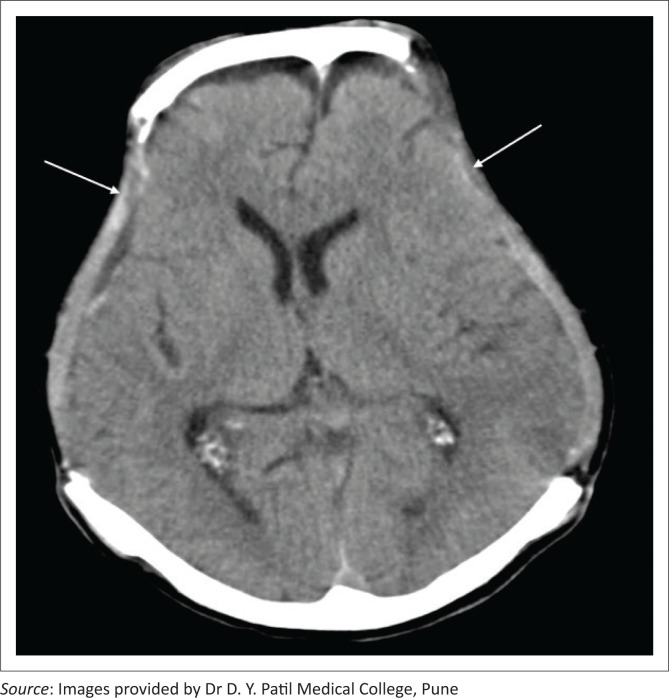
Axial soft tissue window CT image reveals bilateral fronto-parietal craniectomies with sinking of the skin flaps on both sides (left > right) (white arrows) and midline shift towards the right side.
